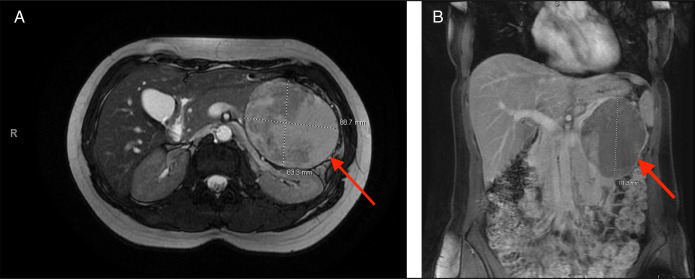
(A) Magnetic resonance imaging (transverse cross-sectional view) of original pancreatic solid pseudopapillary epithelial neoplasm (red arrow). (B) Magnetic resonance imaging (coronal cross-sectional view) of original pancreatic solid pseudopapillary epithelial neoplasm (red arrow)
